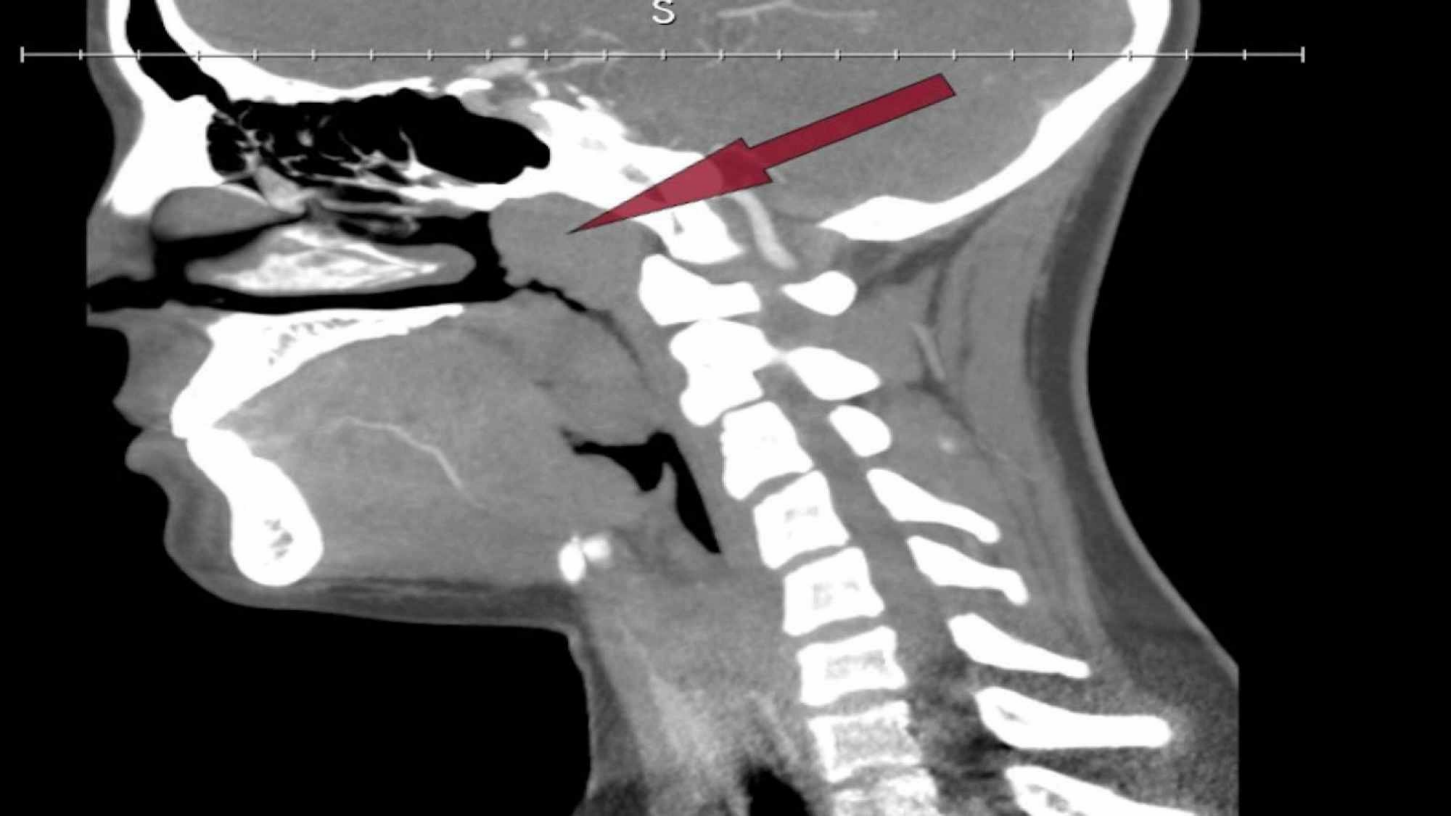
CT angiogram of the head and neck showing enlargement of the nasopharyngeal and oropharyngeal lymphoid tissue.
